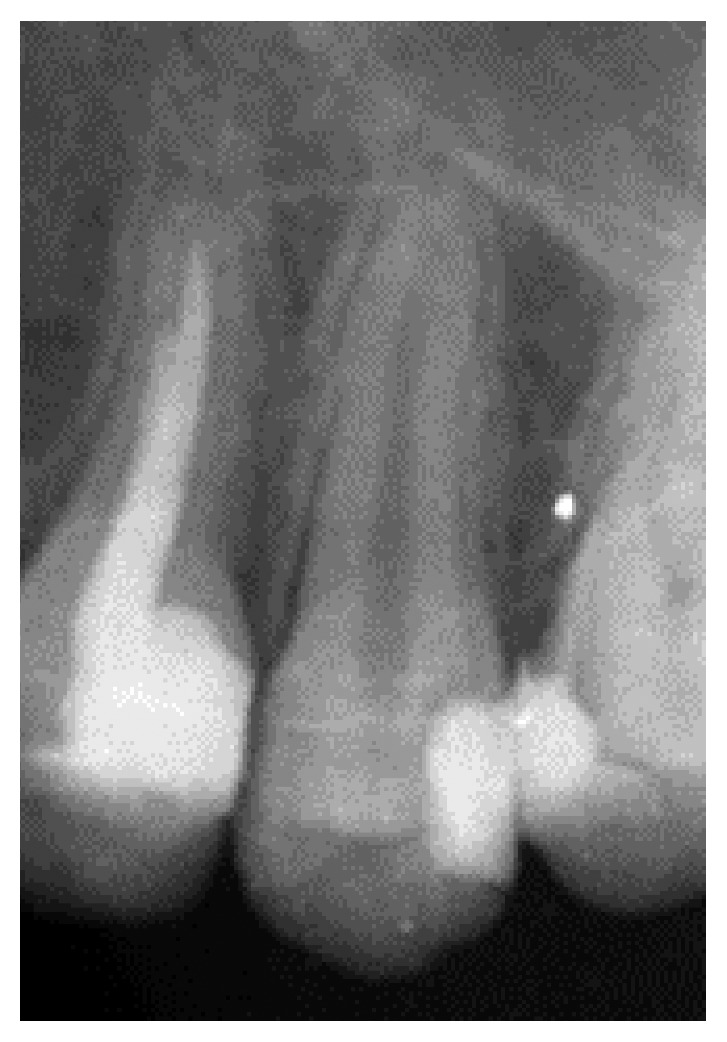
Preoperative radiograph showing a filling shadow close to the pulp and a fully developed root and closed apex of tooth #13.
